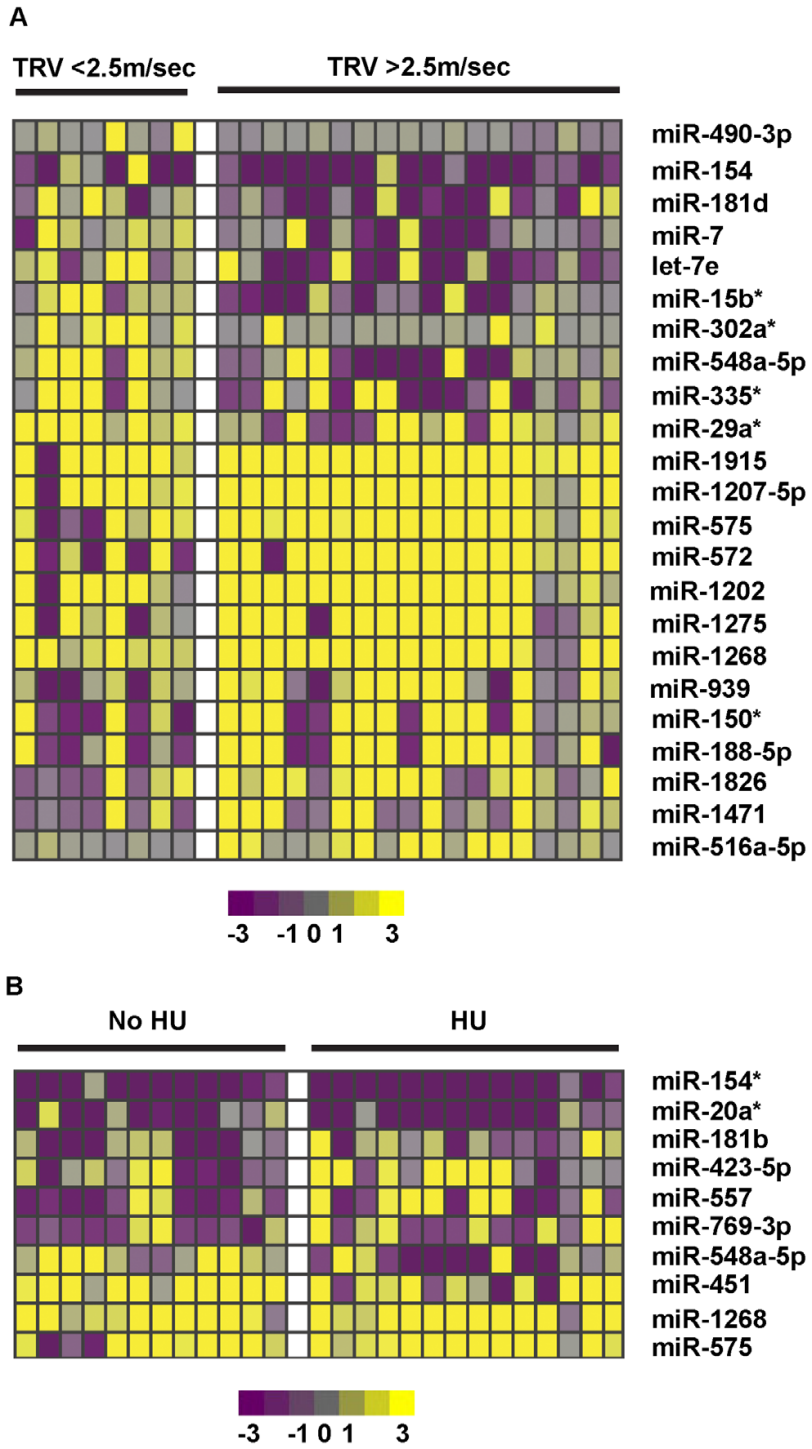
miRNAs in platelets have a unique expression profile in SCD patients based on Doppler-estimated TRV and HU treatment. (A) The heatmap represents 23 statistically significant (*p*-value<0.1, FC>2) differentially expressed miRNAs between subjects with (N = 18) and without (N = 8) elevated TRV (>2.5 m/sec). (B) The heatmap represents 10 statistically significant (*p*-value<0.1, FC>2) differentially expressed miRNAs between the subjects on (N = 14) and off HU (N = 12). Every row represents a gene and every column is a patient. Upregulated miRNAs are shown in yellow color, downregulated miRNAs are shown in purple and no difference is grey. The names of the miRNAs are displayed to the right of heatmap.
